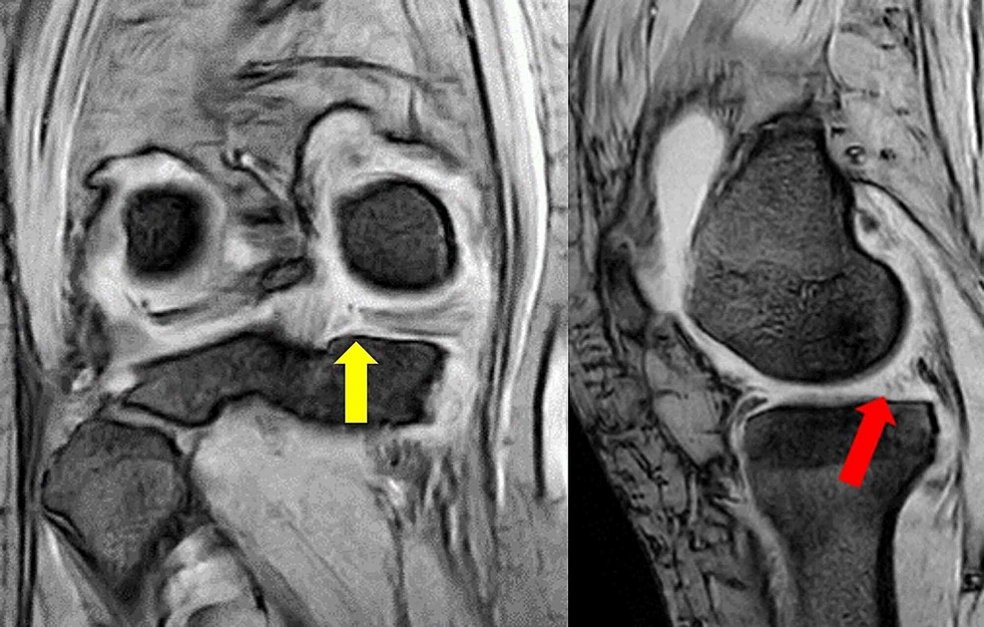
Preoperative MRI T2* (coronal and sagittal views) The images showed truncation sign (yellow arrow) and ghost sign (red arrow) MRI: magnetic resonance imaging
